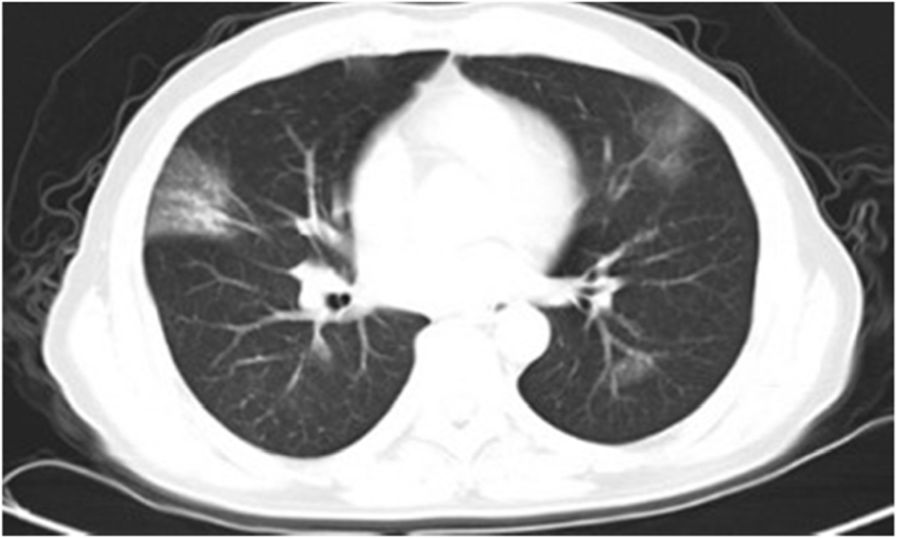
CT imaging of case 2 infected with SARS-CoV-2 on February10, 2020, Illness day 9
